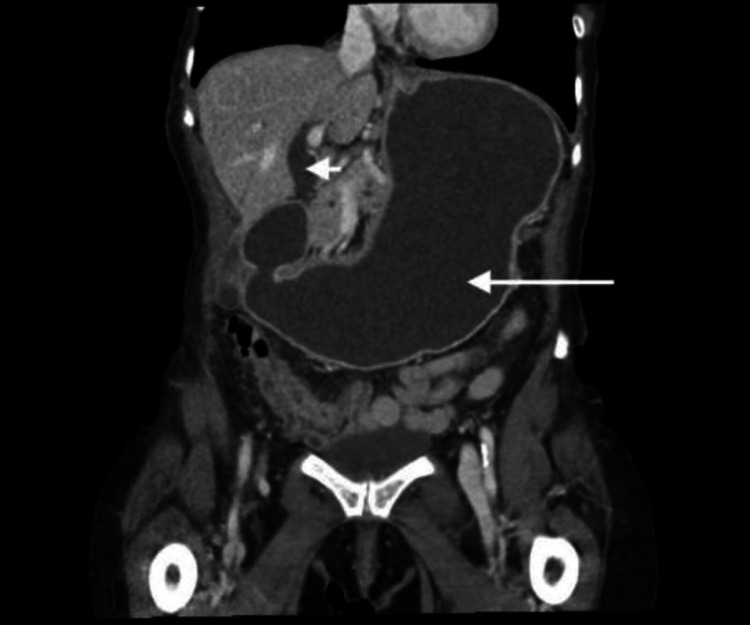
CT abdomen and pelvis with contrast revealing moderate to severely distended stomach (white arrow) with abrupt caliber change in the proximal duodenum. There is also moderate dilatation of the common bile duct (arrowhead) with abrupt caliber change at the ampulla, likely related to the duodenal findings. CT: computed tomography.
